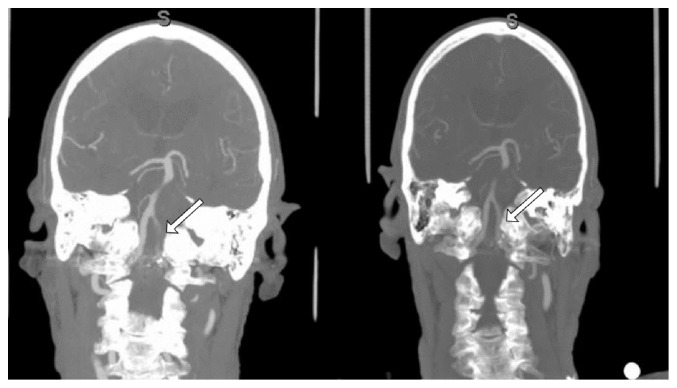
CTA neck coronal MIP planes, (left panel) showing intraluminal thrombus of V4 segment of left vertebral artery (arrow) before cerebral angiogram. Right panel showing patent V4 segment of left vertebral artery (arrow) with resolution of previously seen thrombus.
